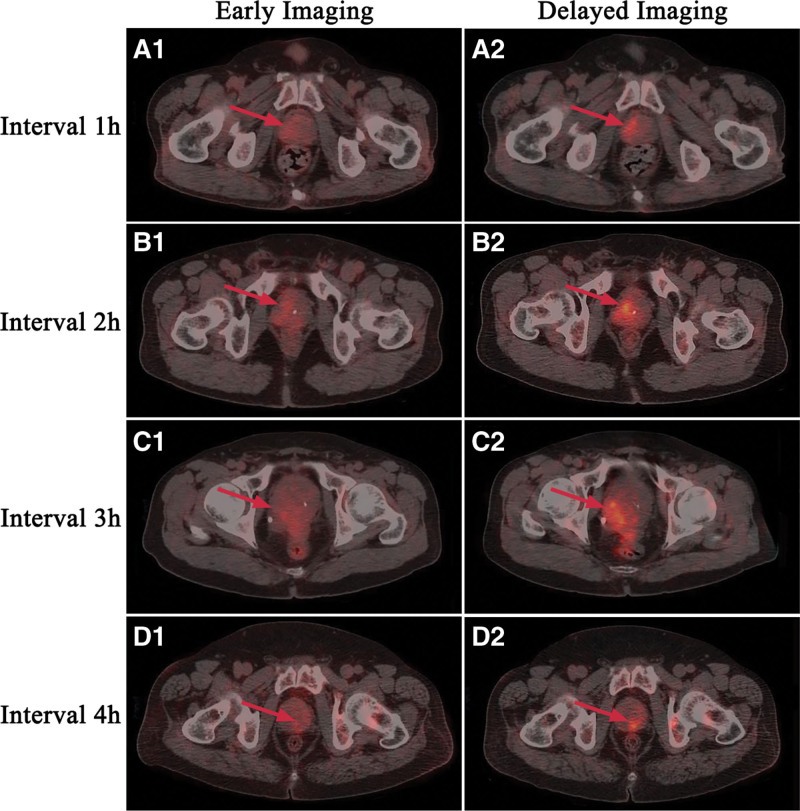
PET/CT imaging of patients with prostate malignant lesions early imaging and delayed imaging after dieresis. (A) The SUVmax of early imaging and delayed 1-hour imaging after diuresis were 3.8 and 4.9, respectively. (B) The SUVmax of early imaging and delayed 2-hour imaging after diuresis were 4.0 and 8.7, respectively. (C) The SUVmax of early imaging and delayed 3-hour imaging after diuresis were 4.1 and 6.7, respectively. (D) The SUVmax of early imaging and delayed 4-hour imaging after diuresis were 3.7 and 5.9, respectively. PET/CT = positron emission tomography/computed tomography, SUVmax = maximum standard uptake value.
